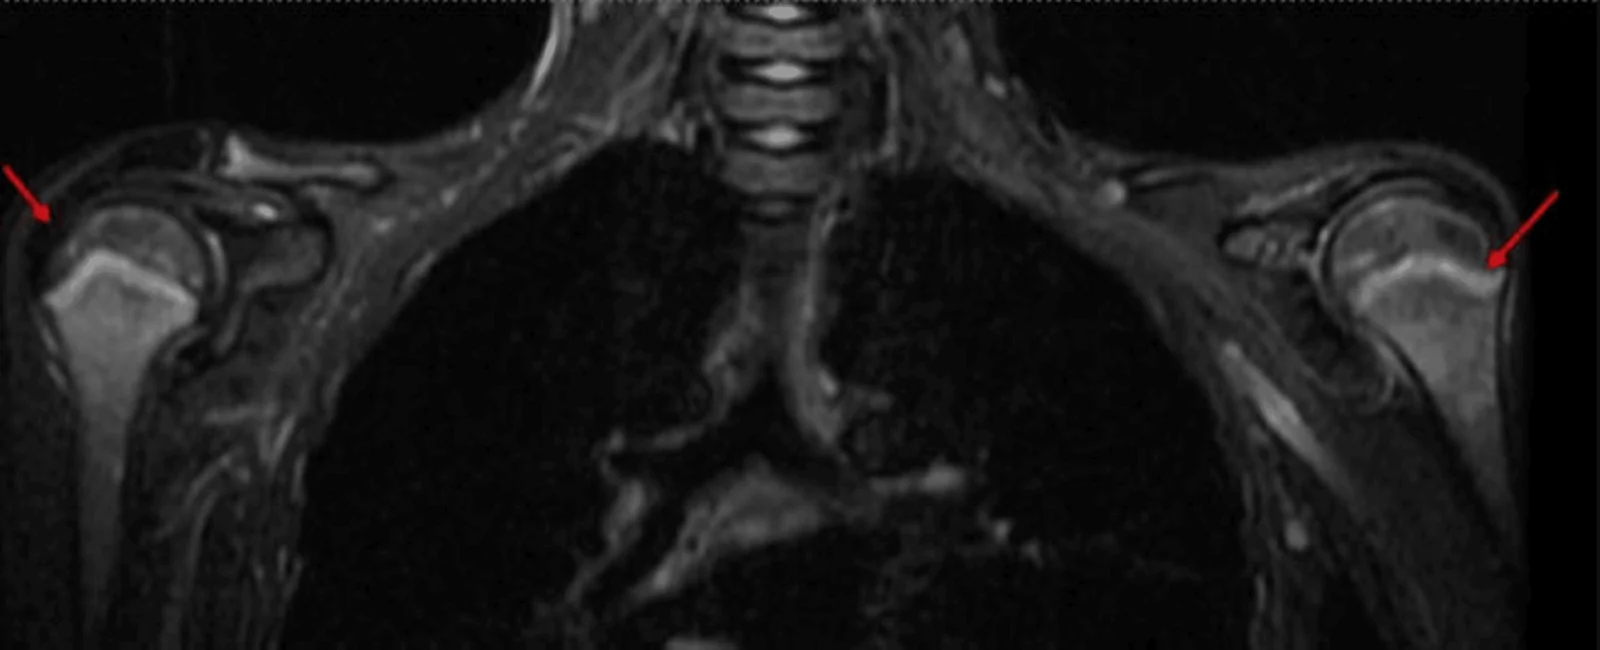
Coronal short tau inversion recovery (STIR) MRI of both shoulders demonstrating symmetric bilateral metaphyseal marrow hyperintensity (arrows) involving the proximal humeri, findings that initially raised concern for chronic recurrent multifocal osteomyelitis.
